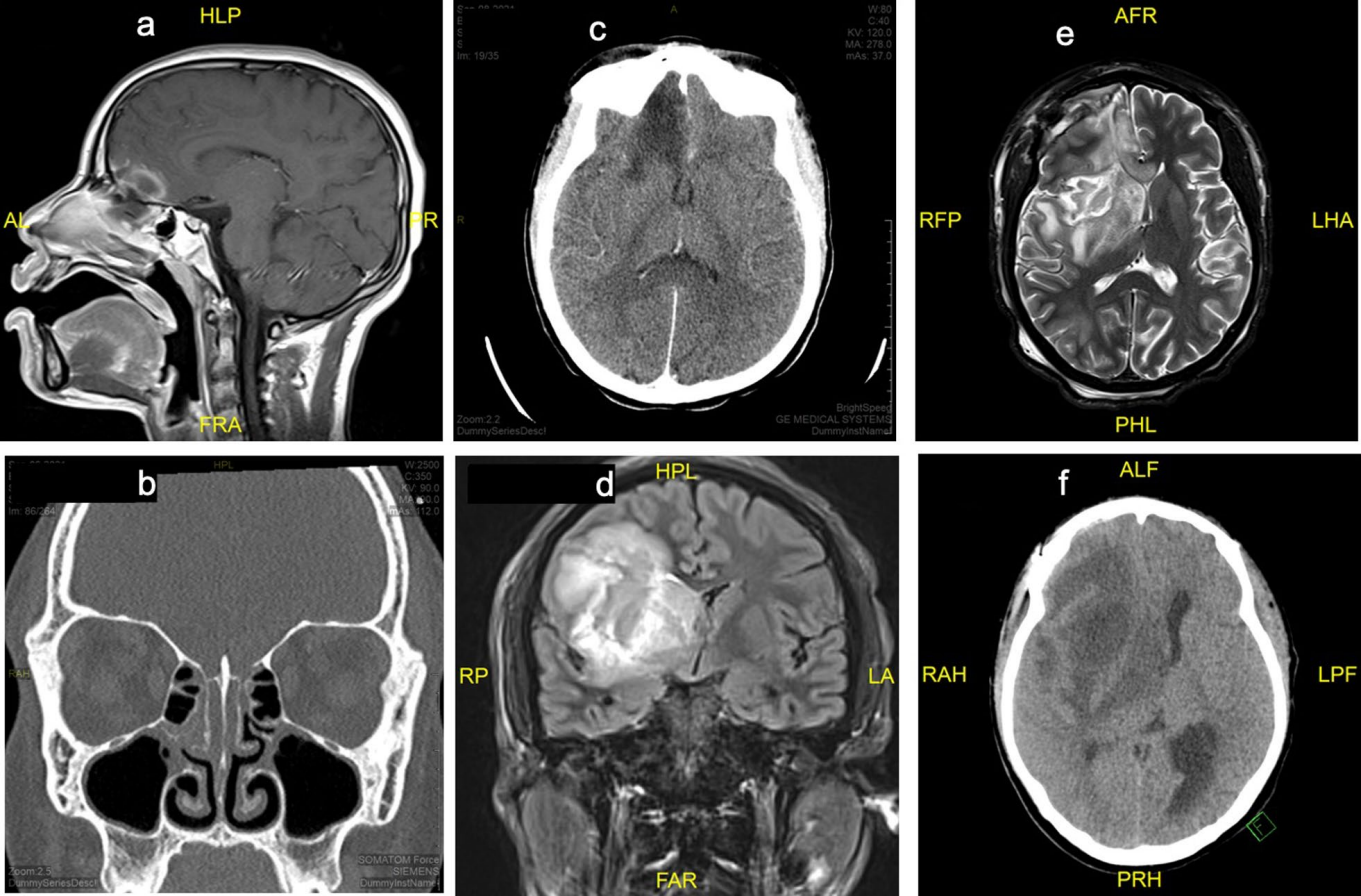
Head images (CT and MRI). a (Day 2 admission): MRI T1 brain sagittal view showed abnormal enhancement within the frontal lobe extending from the olfactory floor upward through the right gyrus rectus. b (Day 3 admission): sinus CT showed mild opacification of superior maeti, maxillary ostium, medial and posterior ethmoid air cells with erosive changes of the osseous septa and possible defect. c (Day 4 admission): head CT showed cerebral edema with mass effect suggestive of infectious cerebritis. d (Day 10 admission): MRI Fluid-attenuated inversion recovery (FLAIR) brain coronal view showed significantly increased area of cerebritis/phlegmon involving right frontal lobe extending into the basal ganglia. Interval increased midline shift with extension of vasogenic edema to the left gyrus rectus. e (Day 16 admission): MRI T2 brain horizontal view showed progression of parenchymal mucormycosis involving right frontal lobe, right parietal lobe, and across the corpus callosum to the contralateral side with a 3-mm right-to-left midline shift. f (Day 25 admission, day 15 post ope, 9/29 images): increased mass effect with right-to-left midline shift with interval increased hypodensity involving the right frontotemporal lobes, basal ganglia, and thalamus extending to the right midbrain, representing increased edema or infection
